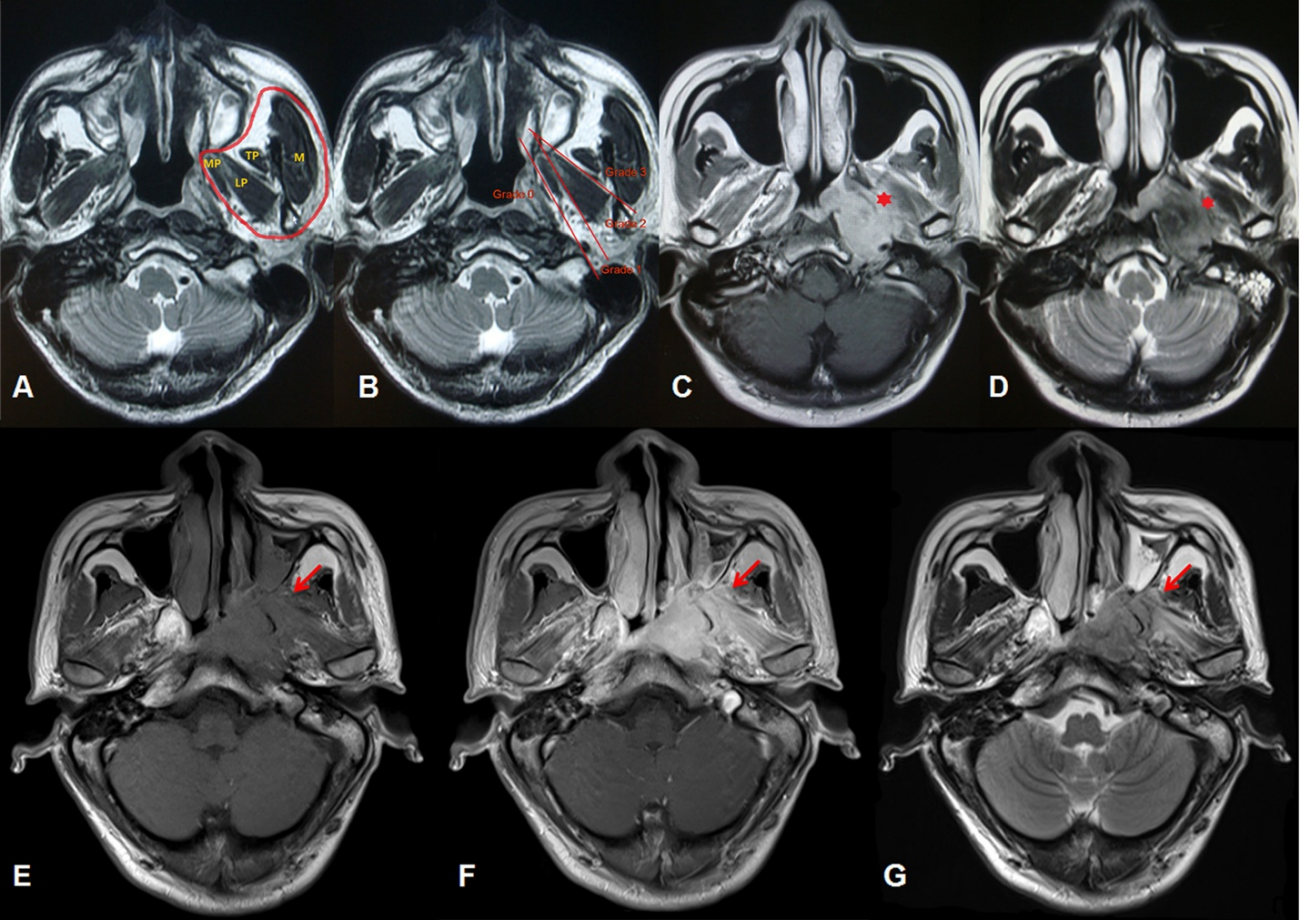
Normal masticator space and different grades of masticator space involvement in magnetic resonance images. (A) Axial T2-weighted magnetic resonance (MR) image (1800 ~ 3000 ms/90 ~ 150 ms, TR/TE) at the level of the nasopharynx shows the anatomic masticator space (circled in red). LP = lateral pterygoid muscle, M = masseter muscle, MP = medial pterygoid muscle, TP = temporalis muscle. (B) Grades of masticator space involvement. Grade 0: without MSI; Grade 1: with medial pterygoid muscle involvement but without lateral pterygoid muscle involvement or infratemporal fossa involvement; Grade 2: with medial and /or lateral pterygoid muscle but without infratemporal fossa involvement; Grade 3: with infratemporal fossa involvement. (C) T1-weighted axial contrast medium–enhanced MR image and (D) T2-weighted MR image show extensive tumor infiltration in the left masticator space. Medial and lateral pterygoid muscle involvement is marked with a red asterisk in each image. (E) T1-weighted axial MR image, (F) T1-weighted axial contrast medium–enhanced MR image, and (G) T2-weighted MR image show left infratemporal fossa involvement (red arrow).
